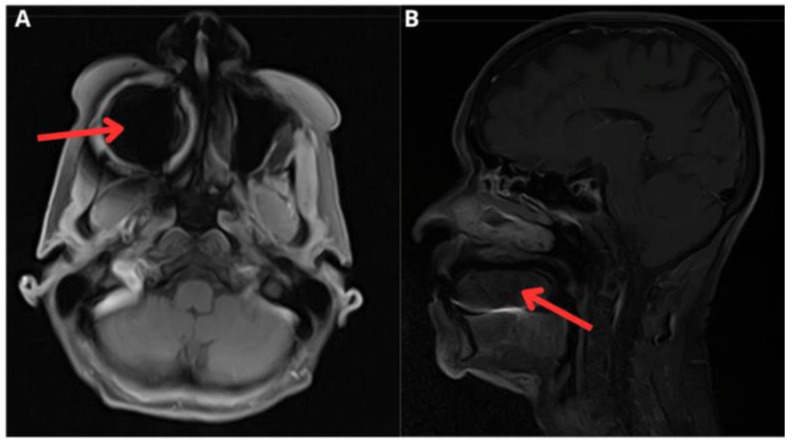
Contrast-enhanced MRI of the face. (A) Axial section. An image of the base of the tongue towards the right side, displacing the muscular structure of the tongue (red arrow). (B) Sagittal section. An ovoid-shaped image with well-defined borders, measuring 41 × 49 × 21 mm, corresponding to a presumably cystic lesion (red arrow).
